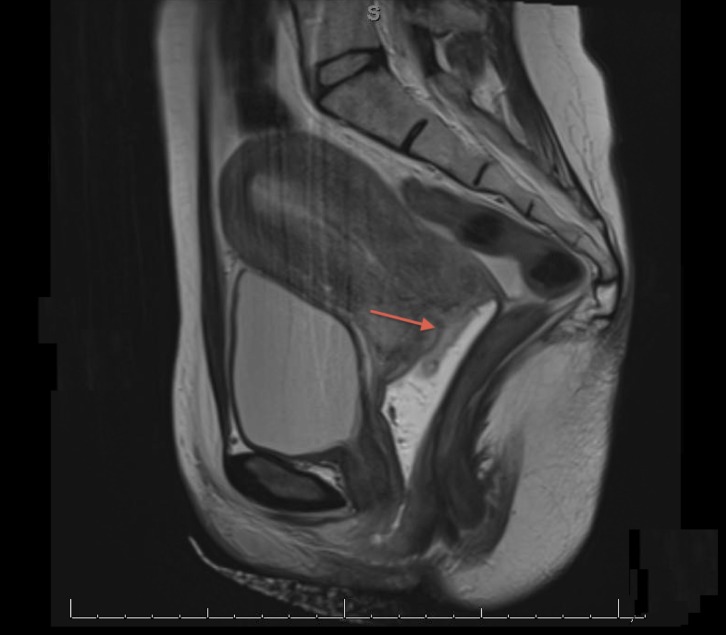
Magnetic resonance image (MRI) of the cervical mass Magnetic resonance imaging, sagittal view. Arrow indicates cervical mass with elevated T2 signal encircling the external cervical os.
